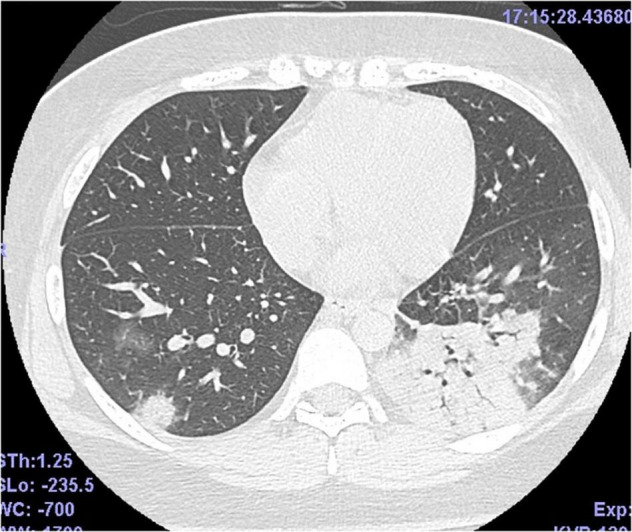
Thoracic CT scan of the mother. Bilateral ground glass opacities and consolidation.
